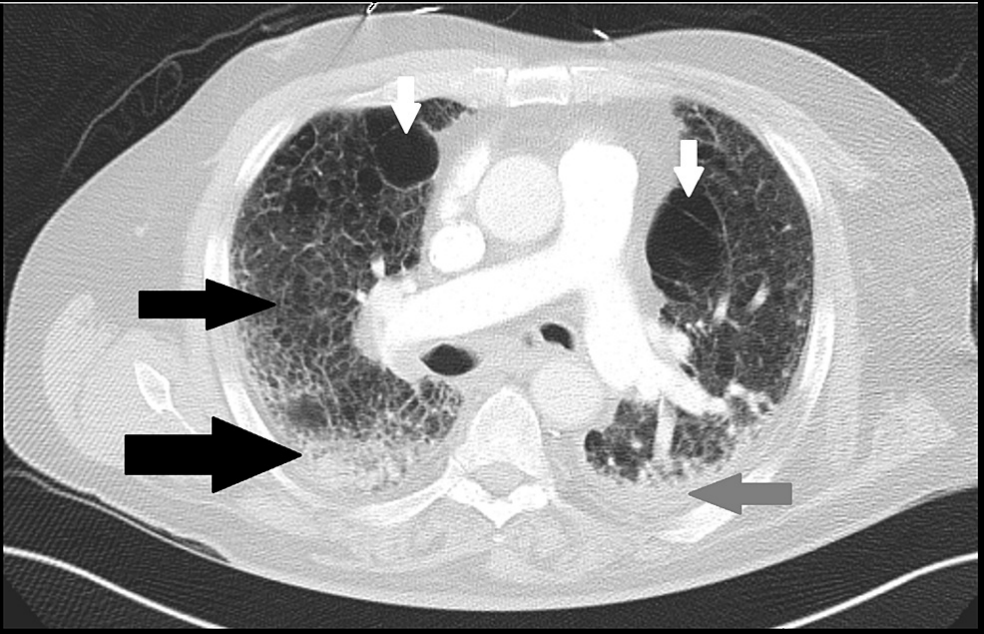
Computed tomography of basilar lungs CT of basilar lungs shows left pleural effusion (grey arrow), pulmonary fibrotic 'honeycombing' pattern (black arrows), residual bullous changes (white arrows), and peripulmonary artery lymphadenopathy.
